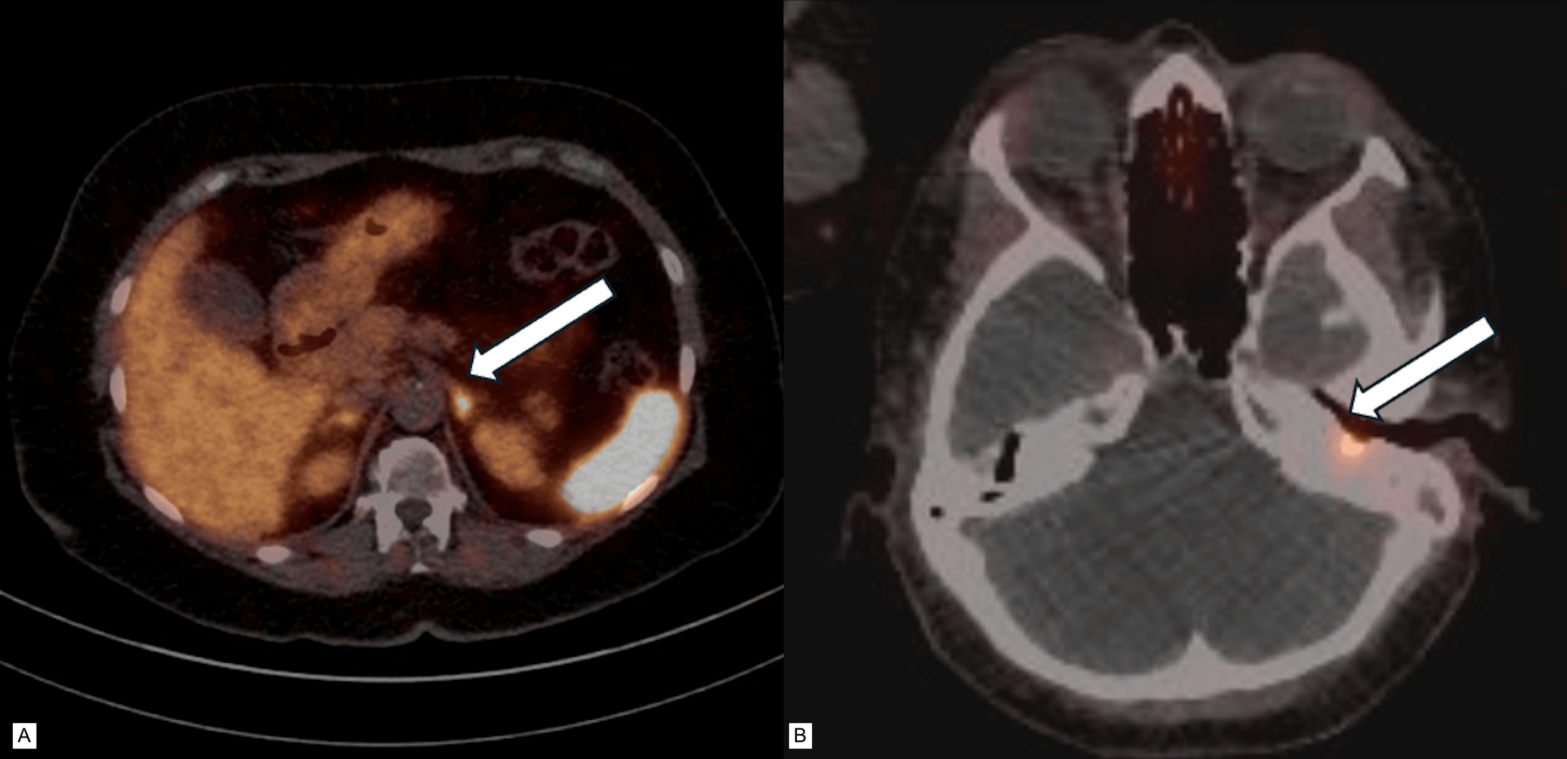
Selected areas of DOTAOC-enhancement from a full-body DOTATOC PET/CT scan in the diagnostic pathway for distant metastasis A: Abdomen: white arrow indicating DOTATOC enhancement of pancreatic corpus. B: Skull: white arrow indicating DOTATOC enhancement of the resection cavity in the middle ear
